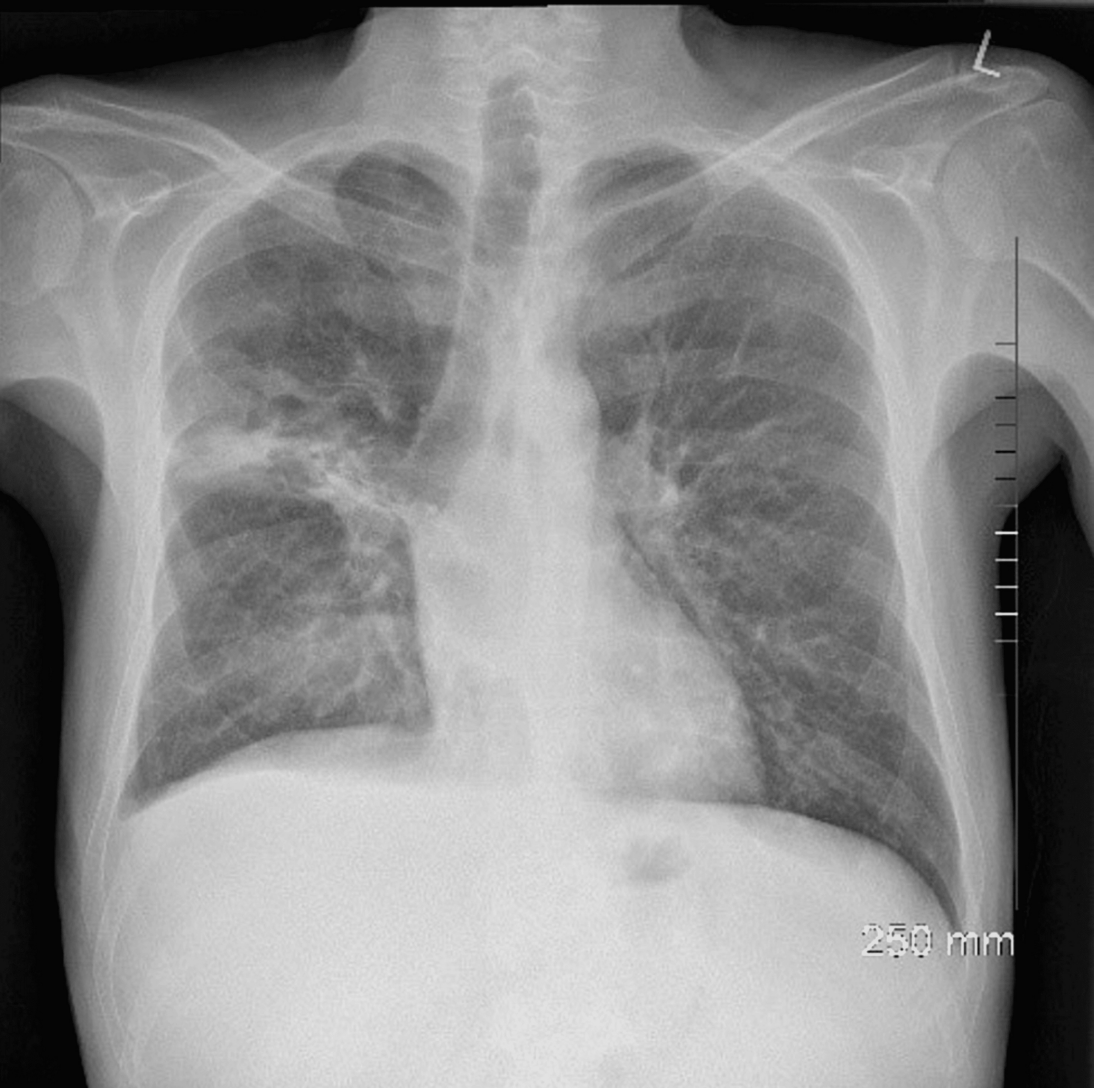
Chest X-ray post treatment
